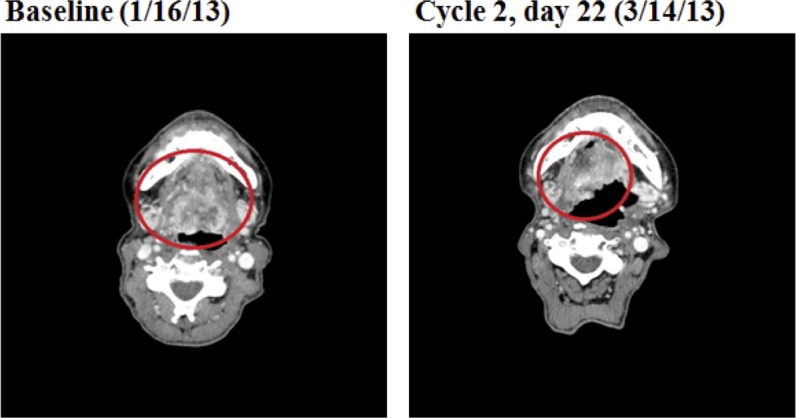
A patient with HNSCC showed partial response (−54%) on the first restaging scan The baseline and the first restaging CT scan of a 60-year-old woman with squamous cell carcinoma of the base of the tongue who had received prior cetuximab.
